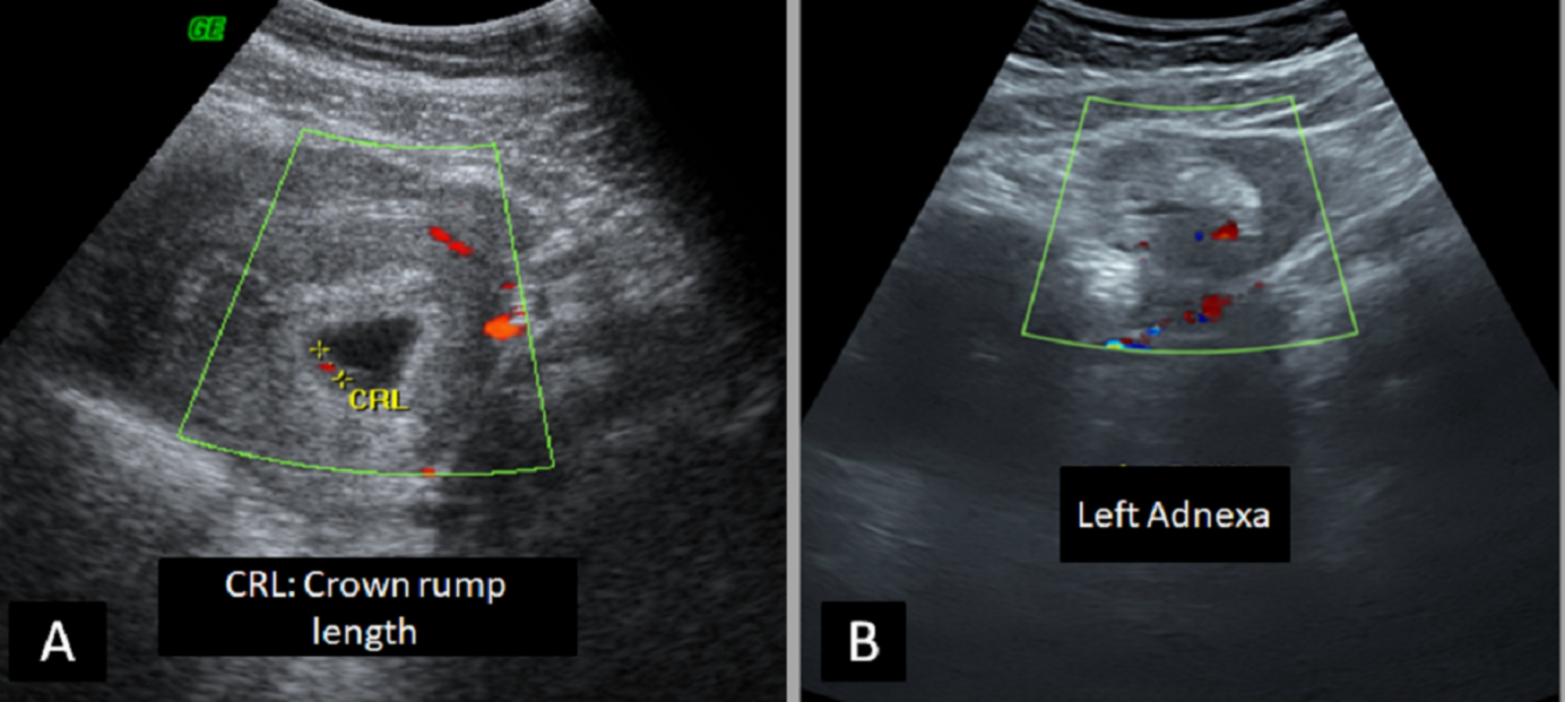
Transvaginal ultrasound A) There is a single viable intrauterine gestational sac; B) a large hematoma with a central, thick echogenic ring is seen, indicative of ruptured left ectopic pregnancy. A right adnexal lesion is also noted. These findings favor the diagnosis of a disturbed heterotopic pregnancy.
